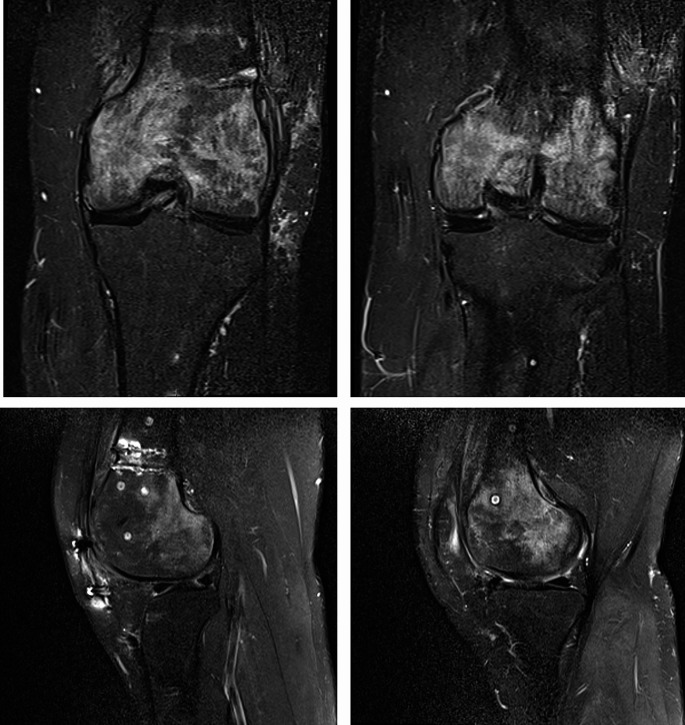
BME at MRI in a 29 year old male after trocheoplasty, femoral varus osteotomy, and anterior tibial tuberosity transposition
